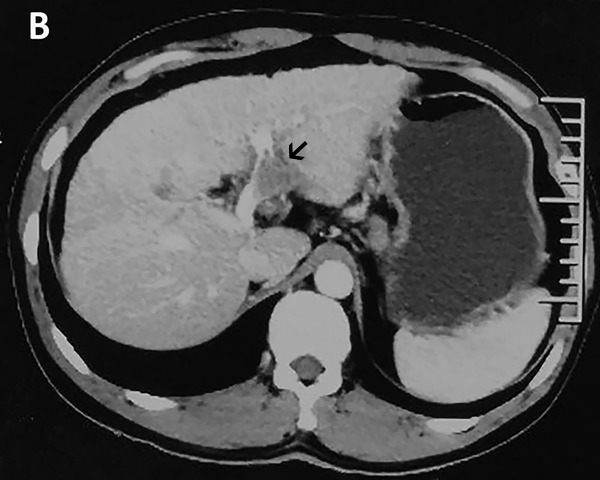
Abdomen CT images show that one of the lesions is located in the top of liver A. In the venous phase, the mass is low density and irregular. B. The arrow represents tumor thrombus in the left branch of portal vein.
